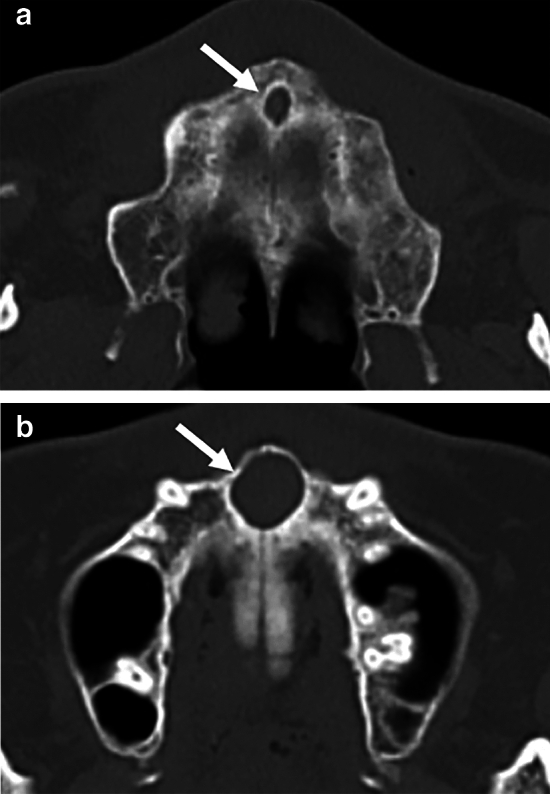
Identification of nasopalatine duct cyst (NPDC) using computed tomography (CT). (a) Axial CT shows normal NPD (arrow). The maximum diameter of NPD is 5.0 mm. (b) Axial CT shows NPDC (arrow). The maximum diameter of NPD is 11.8 mm. If the maximum diameter of NPD was 6.0 mm or more on Axial CT, it was identified as NPDC.
